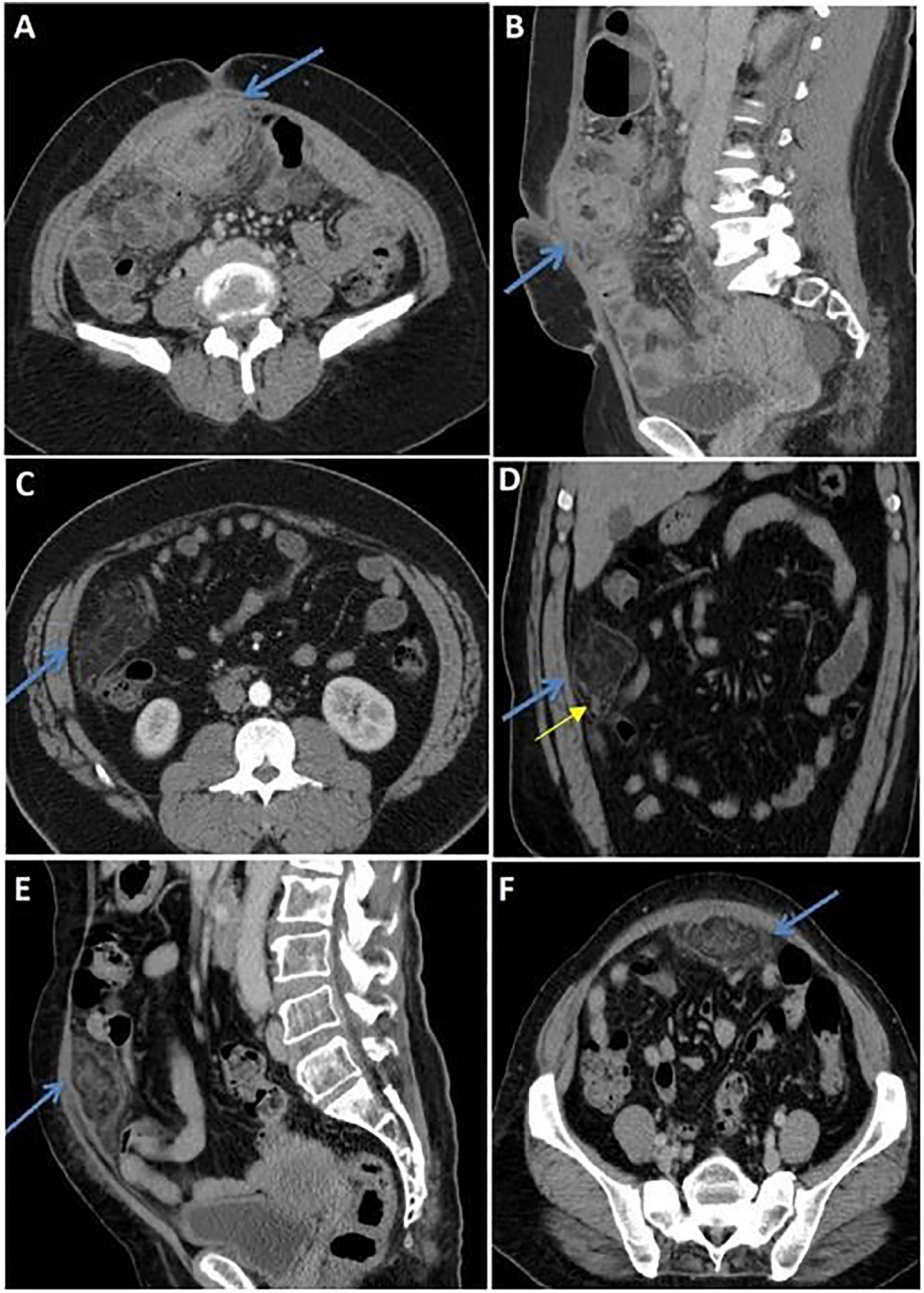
Radiologic findings. (A, B) CT coronal and sagittal view shows a focal soft tissue density area with surrounding fat stranding with omental vessels swirling within. (C, D) CT axial and coronal view showing extensive localised fat stranding lateral to the ascending colon and inferior to the right lobe of the liver with a thin peripheral hyperdenserim. Linear vertical collection of fluid attenuation is seen – omental infarct with a small abscess (yellow arrow). (E, F) CT sagittal and axial views showing an encapsulated omental infarct in the hypogastric region.
